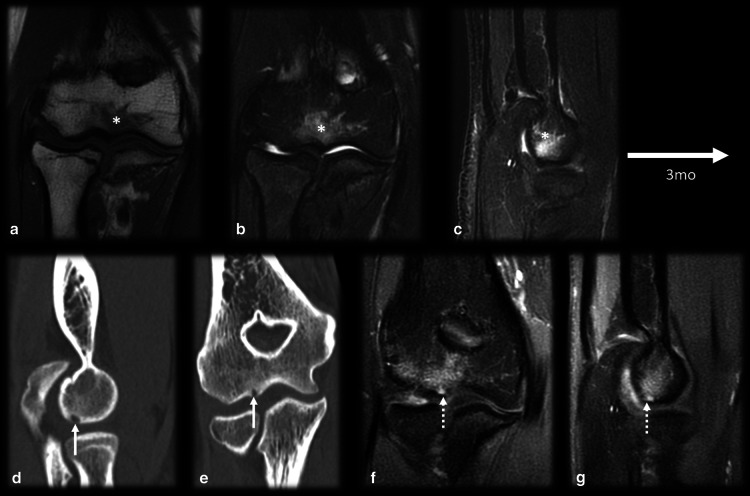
Osteoid osteoma mimicking bone marrow contusion. Male, 16 years old, presenting with right elbow and arm pain for 2 days after falling during physical activity at school. First, the MRI T1 (a) and T2 FS (b, c) findings were interpreted to indicate contusion bone marrow edema (asterisks) without a fracture. However, the pain persisted for more than 3 months. Follow-up CT (d, e) and MRI (f, g) scans revealed small subchondral OO with a lytic nonmineralized nidus (arrows) and enhancement (dotted arrows) on the T1 FS GD MRI scan (f, g)
